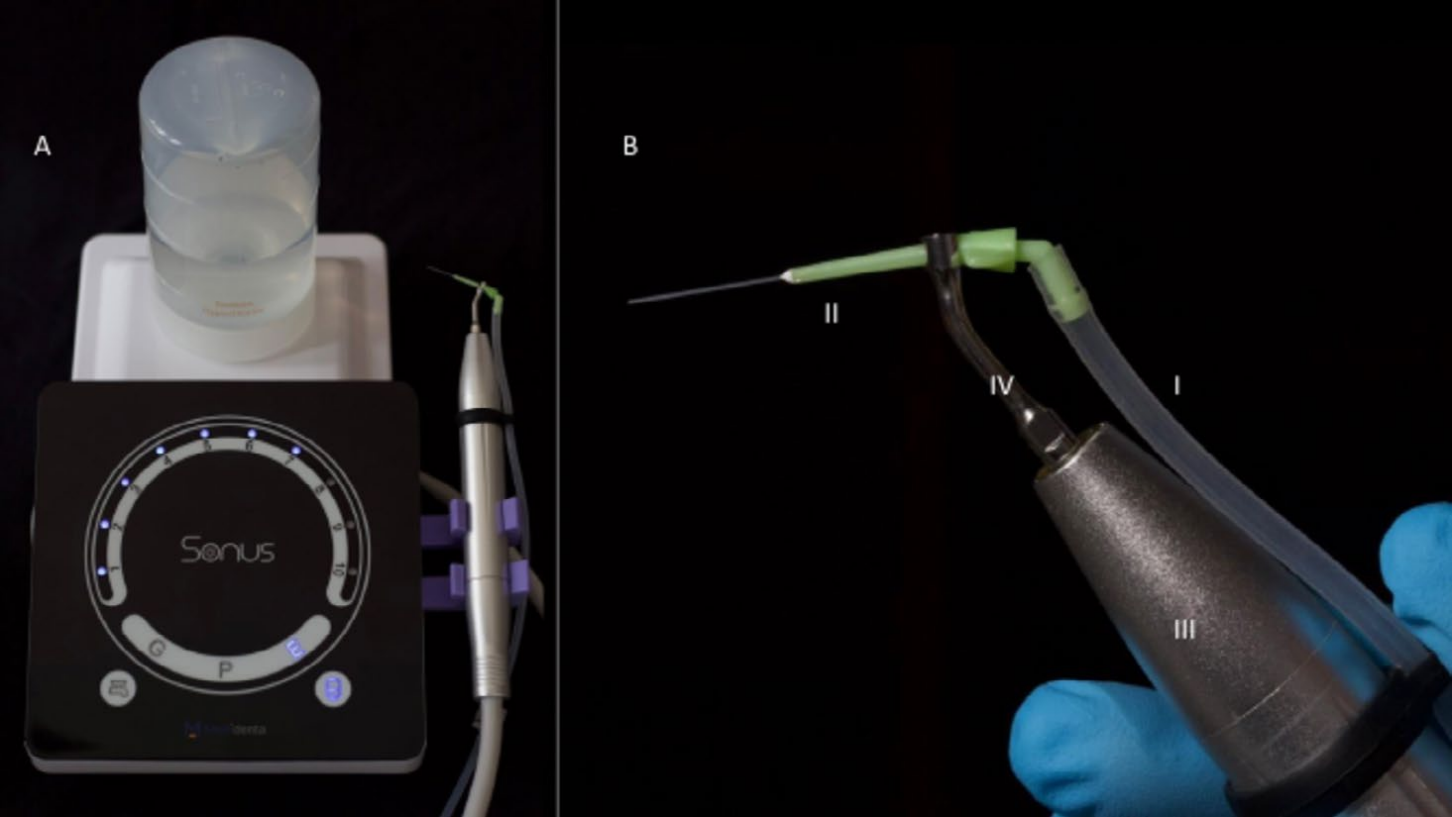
iVac device. (A) Sonus piezoelectric ultrasonic unit with iVac system attached. (B) 0.35‐mm cannula (II) connected to small suction tubes (I) attached to a piezoelectric ultrasonic handpiece (III) through a connector (IV) (S‐type insert).
